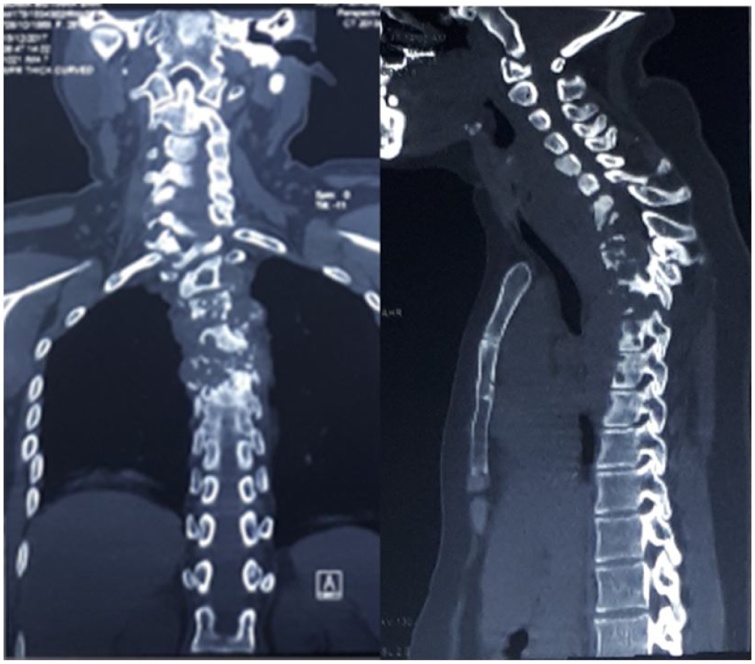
Preoperative CT images demonstrated extensive vertebral body destruction, several facet joint dislocations and mild retrolisthesis of C4 to C5 segment.
